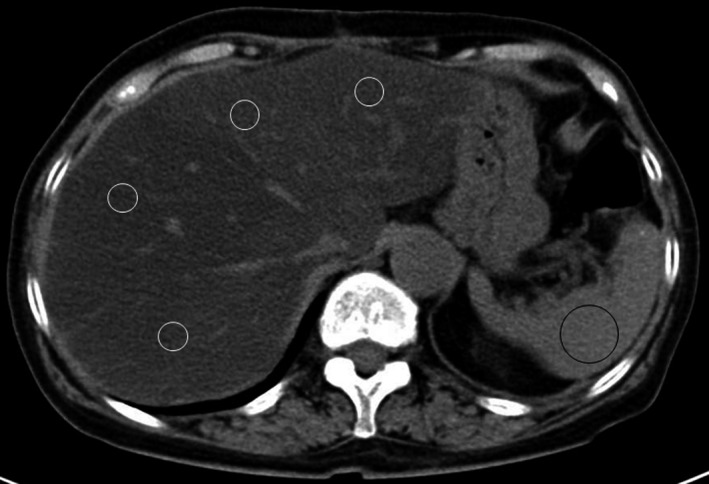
We measured the average computed tomography (CT) attenuation values in four sectors of the liver and the median value from all sections of the spleen on unenhanced CT scans. Nonalcoholic fatty liver disease was defined as a liver‐to‐spleen attenuation ratio of <0.9 or a hepatic attenuation value at least 10 Hounsfield units lower than the spleen attenuation value.
